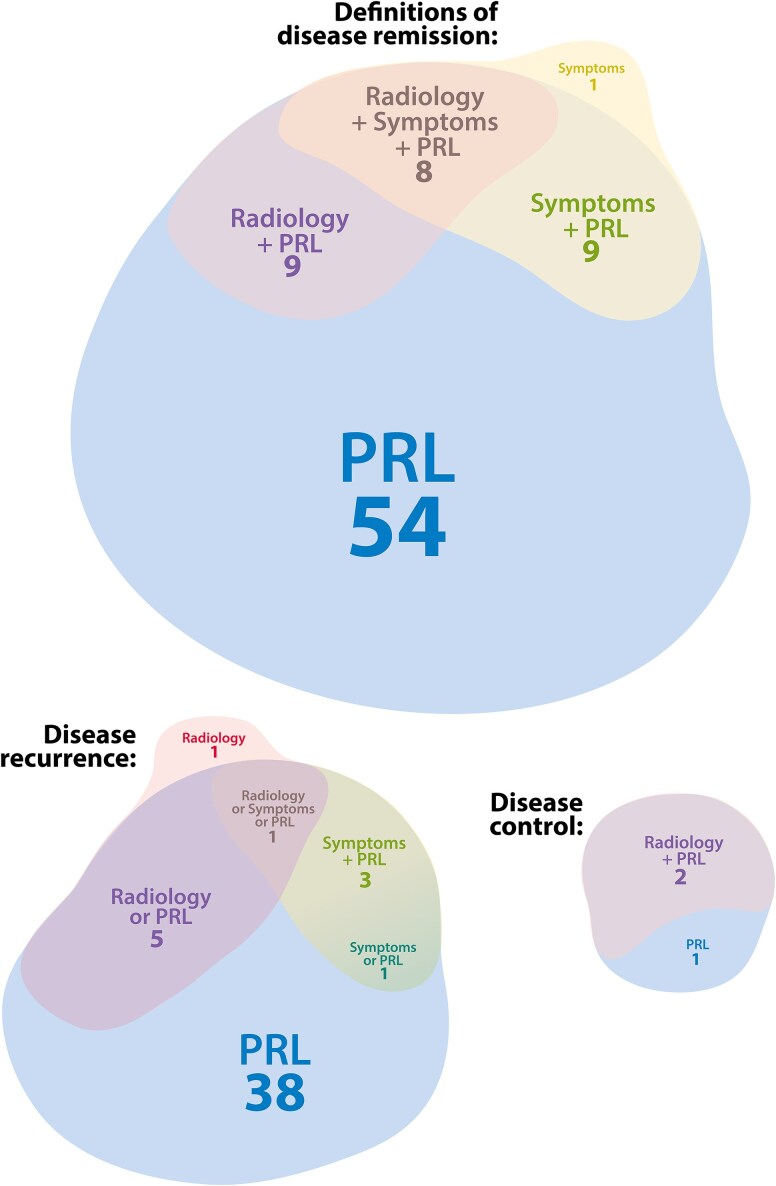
Visual representation of parameters used in the definitions of *disease remission*, *disease recurrence,* and *disease control* as reported in literature. The number of studies using the specific parameters in their definition is provided. Sixty-three studies reported a total of 81 definitions of remission, among which 27 were unique definitions. Forty-six studies reported 49 definitions of disease recurrence, among which 20 unique definitions, and 3 studies reported 4 unique definitions of disease control. “Symptoms” concern clinician-reported symptoms. “Radiology” is magnetic resonance imaging of the pituitary. Abbreviation: PRL, serum prolactin.
